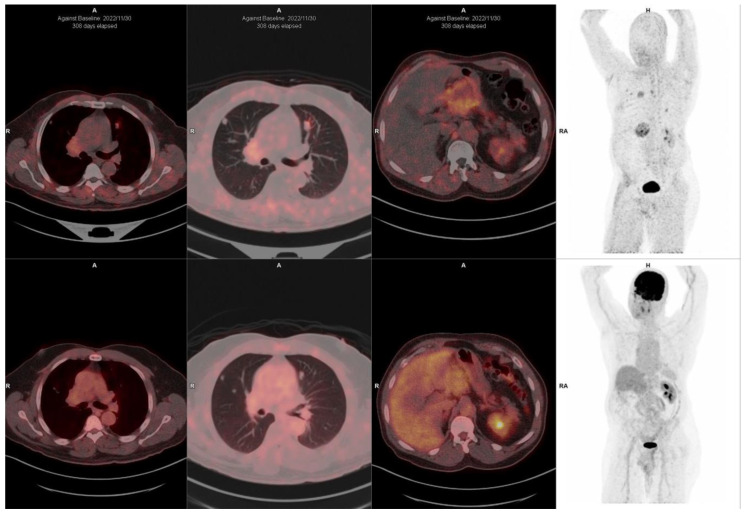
65-year-old female with renal cell carcinoma post right nephrectomy who presented with a mass in the region of the head of pancreas. ^68^Ga-FAPI-46 (upper panel) shows avidity in the head of pancreas mass, right hilar lymph node, and the lung compared to ^18^F-FDG (lower panel). These lesions are better seen on the FAPI-46 PET images due to the better tumor-to-background ratio.
